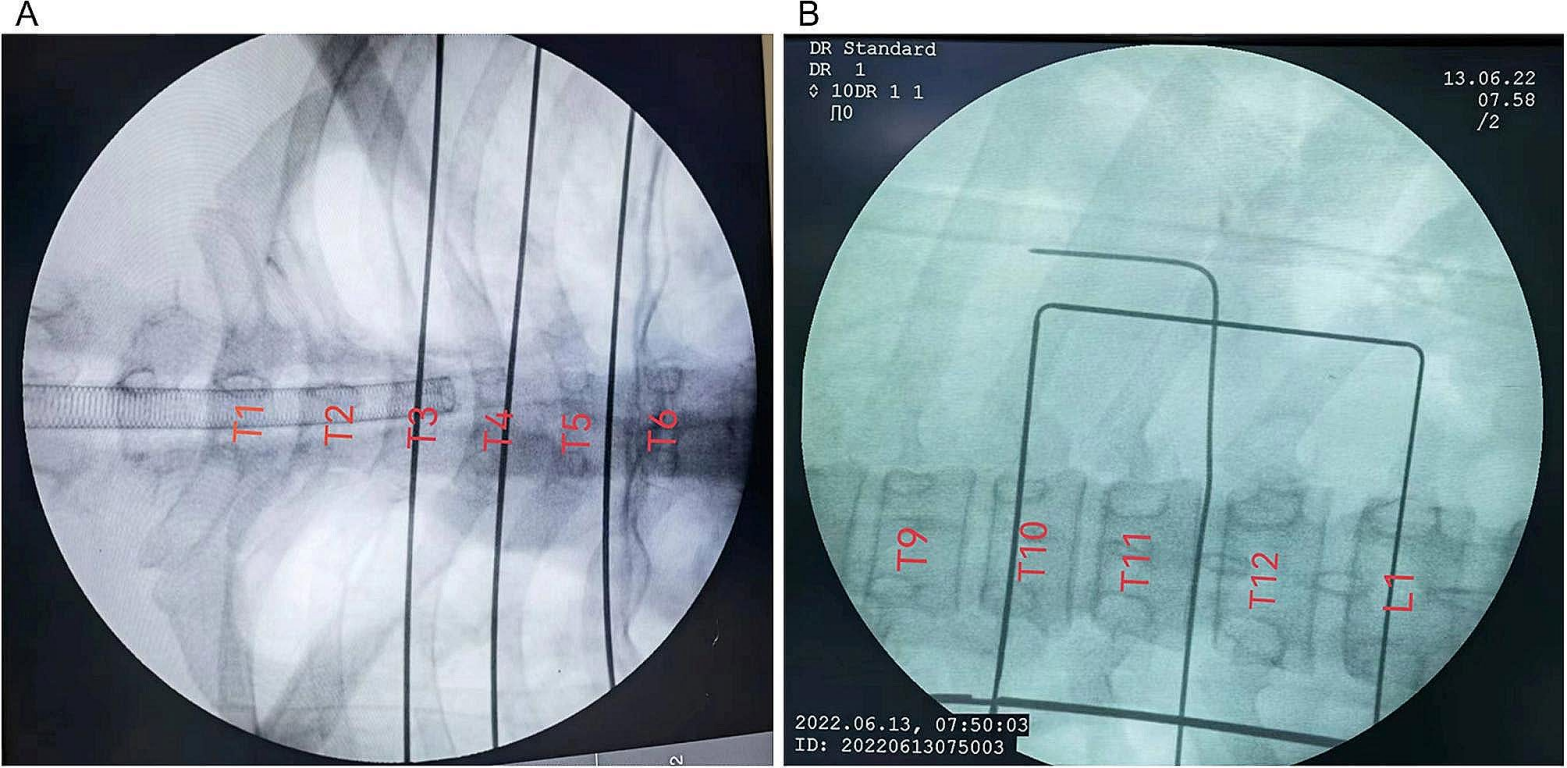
A and B: Using metal needles, the T1–T12 thoracic vertebral body segments were marked with a C-arm. Each X-ray image had two neighbouring vertebral body metal markers for tumour localization
